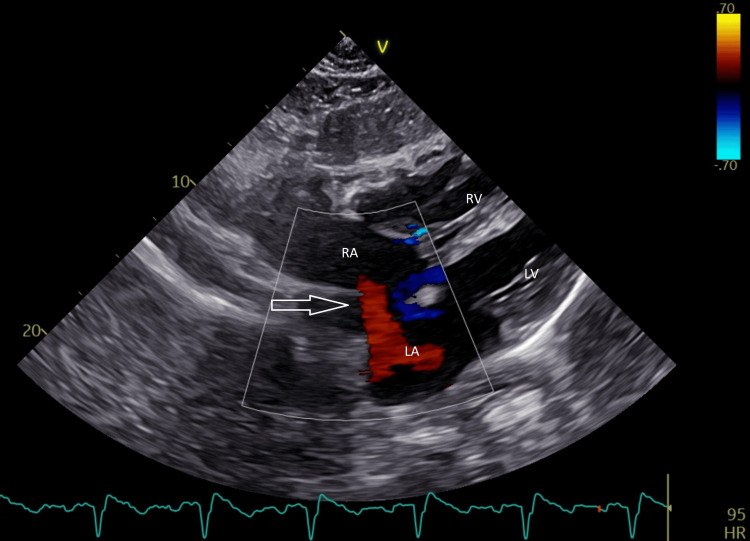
Transthoracic US with color Doppler demonstrating ASD (arrow) with bidirectional shunt LA: left atrium; RA: right atrium; RV: right ventricle; LV: left ventricle; ASD: atrial septal defects; US: ultrasound
